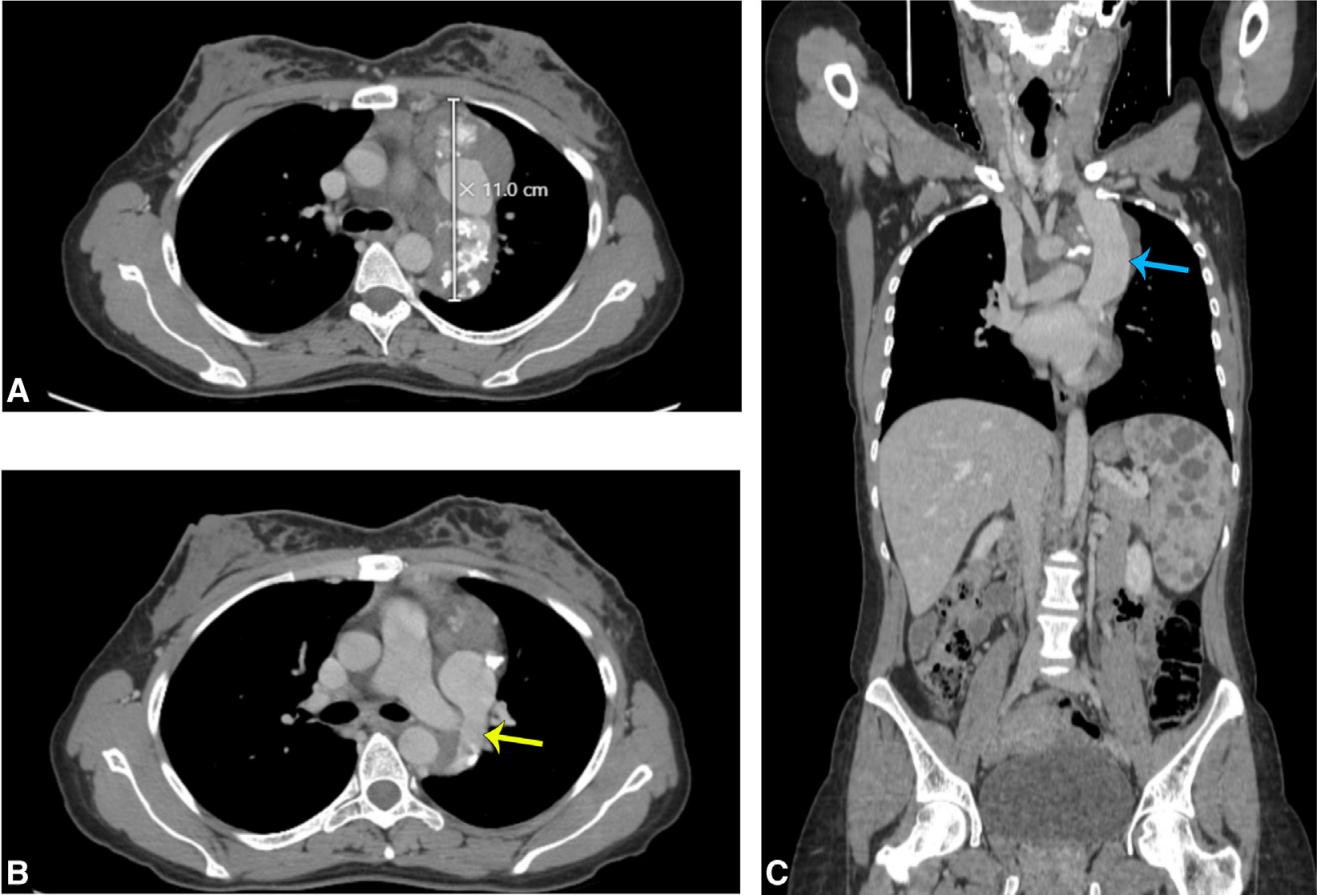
Venous phase of preoperative computed tomography scan. A, The lesion at its widest extent. B, The main feeding vessel of the hemangioma originating from the left superior vena cava (*yellow arrow*). C, Sagittal view showing the dilated left superior vena cava (*blue arrow*). Diffuse lesions of the spleen can be seen.
